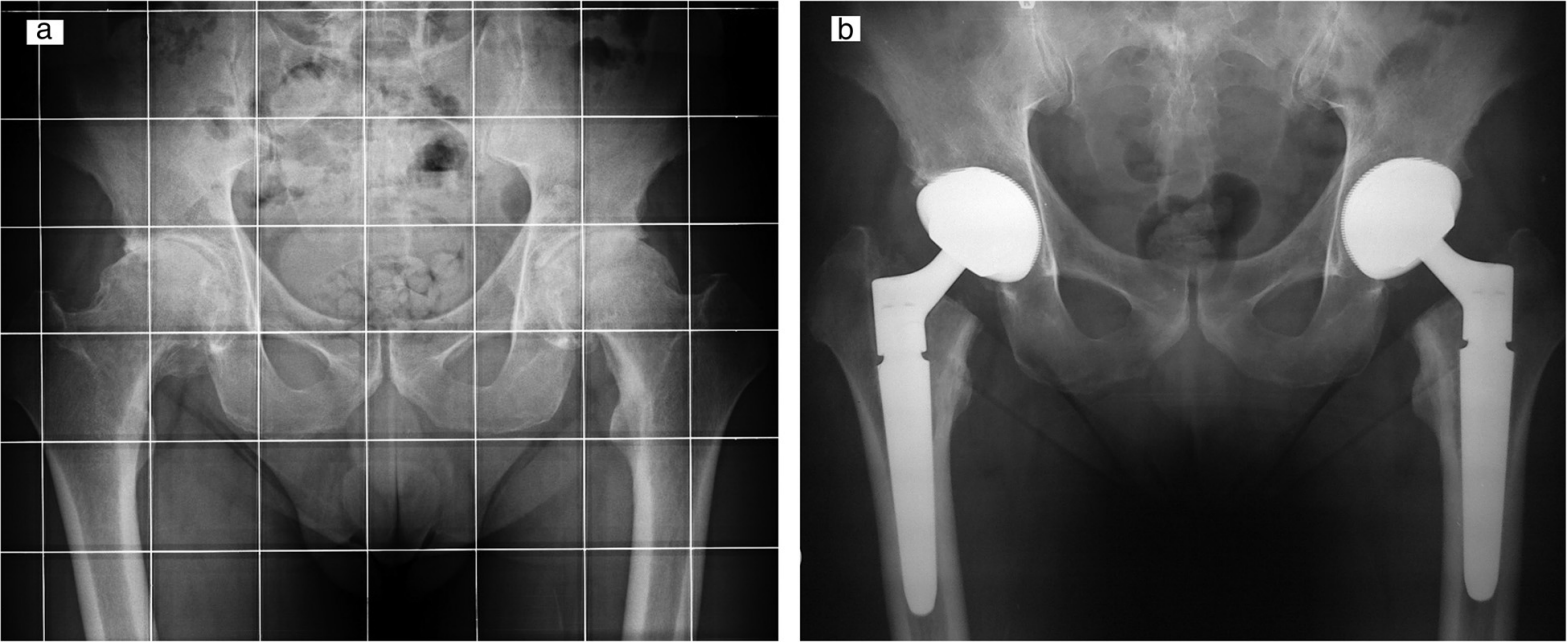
Bilateral hip osteoarthritis in young patient: X-ray pre-operatory (a) and at 3-year follow-up (b)
